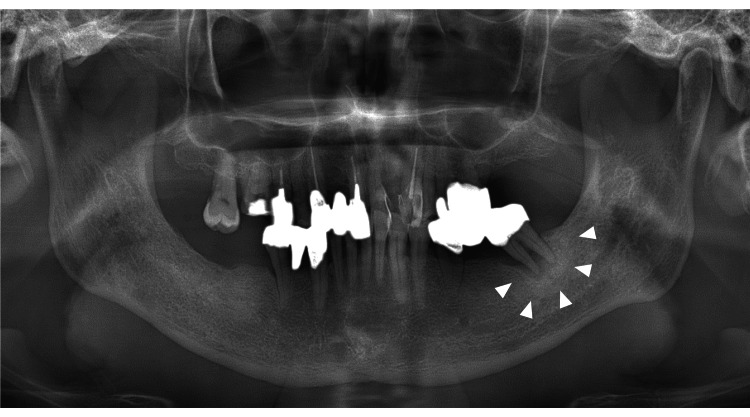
Panoramic radiograph Diffuse osteosclerosis equivalent to 37 was observed, and horizontal bone resorption of alveolar bone in the remaining tooth was moderate.
